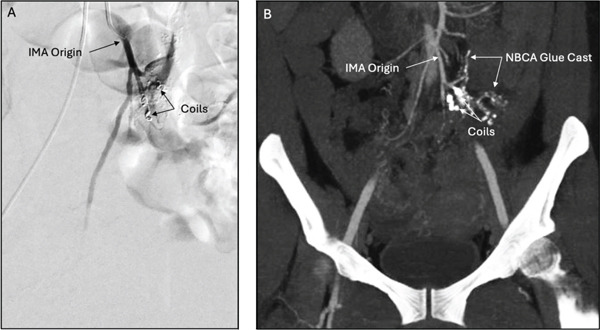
Postembolization imaging. (A) Final IMA angiography demonstrates coils within the sigmoid and descending left colic branches with resolution of flow through the AVF and no active extravasation. (B) Coronal CT angiography at 8 months postprocedure confirms complete occlusion of the IMAVF. Coils and NBCA glue cast are identified without evidence of recanalization.
